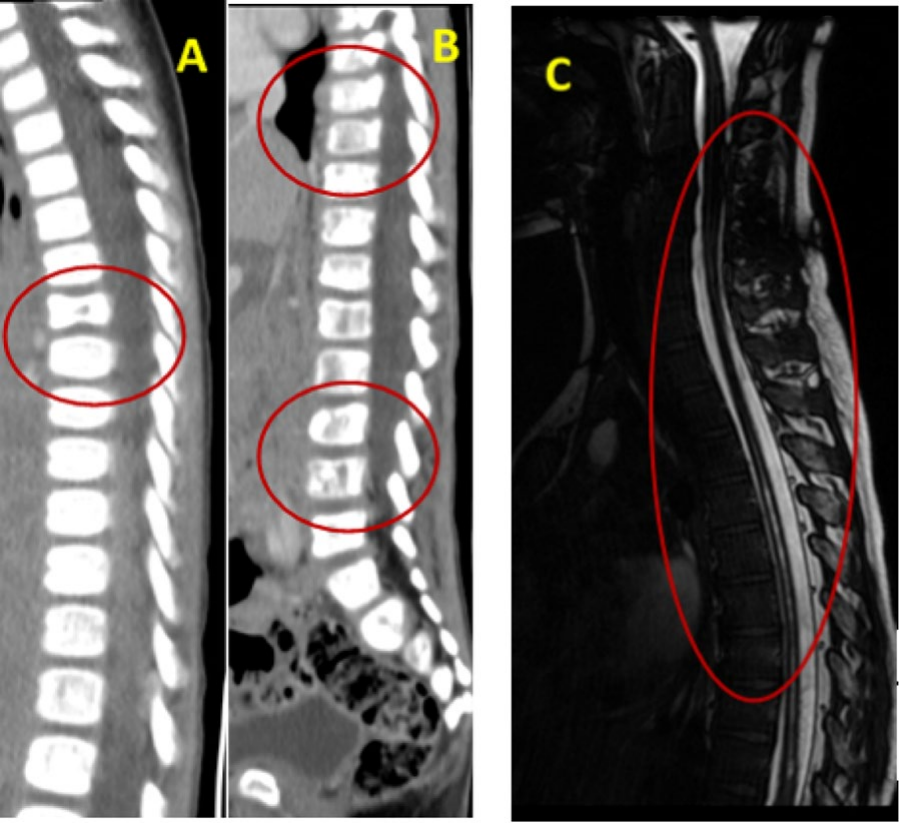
(A–C). Osteolytic lesions marked with red circles. Figure A shows child 5, Figure B shows child 6, who presented with symptoms of spinal cord compression and paraplegia. CT scans revealed extensive metastatic lesions over the vertebral body. These lesions were lytic lesions with a surrounding zone of sclerosis. Bone marrow biopsy revealed a diagnosis of neuroblastoma.Figure C–Child 7 presented with infantile neuroblastoma with extensive vertebral metastasis and hydromelia. MRI revealed lesions and cord involvement. Bone marrow biopsy revealed a diagnosis of neuroblastoma
